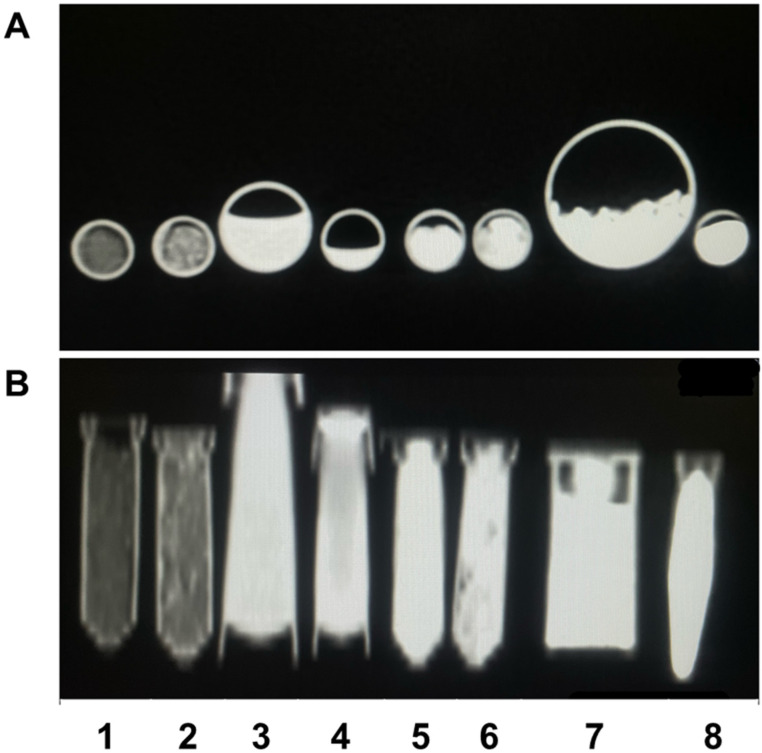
CT imaging showing axial section (A) and coronal section (B) of the samples Zn-HA 10% (1), Sr-HA 10% (2), HA 282 STT (3), HA 212 500 °C (4), HA 01.256 CTT 1000 °C (5), HA microspheres (6), AuNP (7), and Cu-GO (8).
